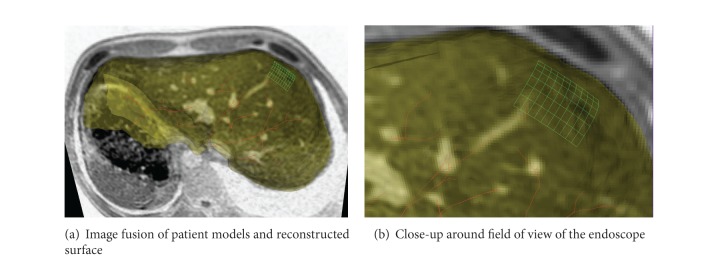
Fusion of patient models and reconstructed surface from the stereo endoscope. Green mesh is the reconstructed surface from two endoscopic images, red curves are the centerlines of vessels, segmented surface from MR images is shown in semitransparent yellow, and background is one MR slice with bright vessels.
